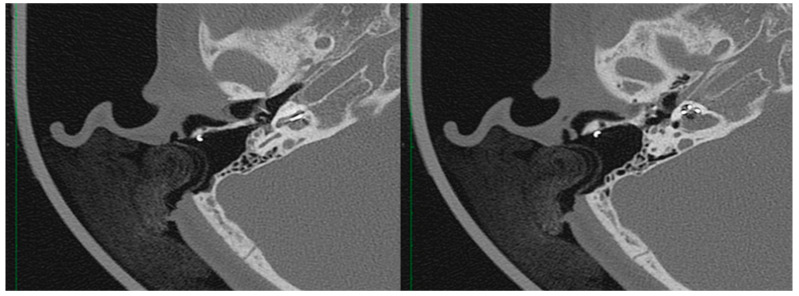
Intraoperative CT scan images of patient 4.
